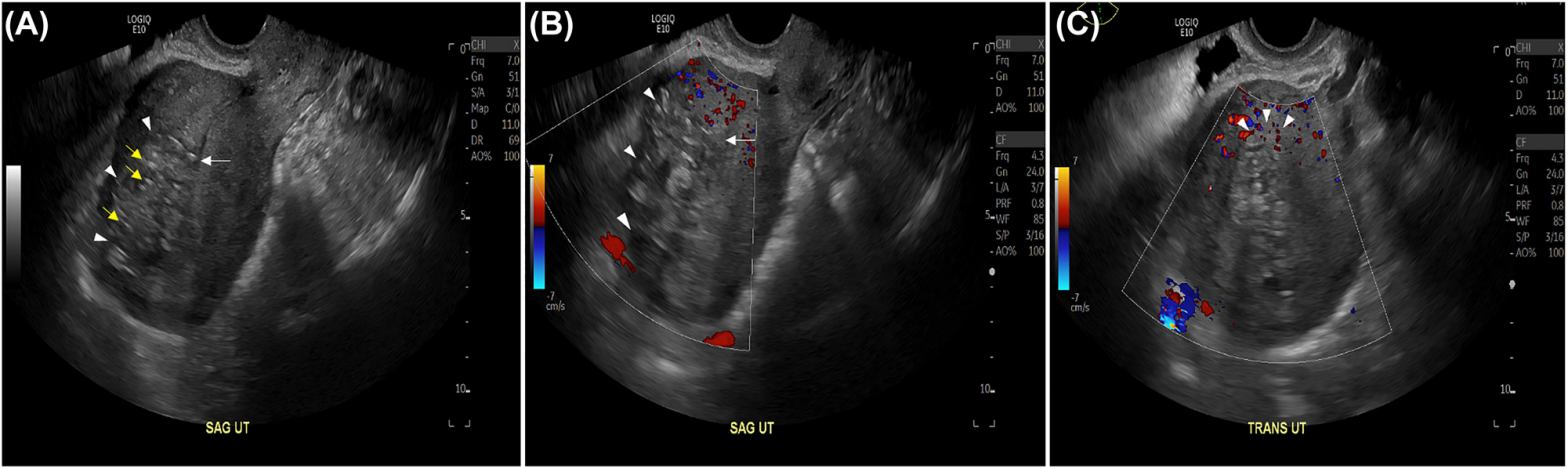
Sagittal (A, B) and axial (C) transvaginal ultrasound images obtained on post-operative day 18. The images demonstrate ill-defined infiltrating echogenicity (white arrow heads) that extends into the subserosal anterior myometrium, with minimal protrusion into the endometrial canal (white arrow), raising initial concern for potential invasive placentation. However, no vascularity is noted within the area on Doppler (B, C). Parallel linear echogenicities (yellow arrows A) are consistent with sutures indicating the hysterotomy site.
